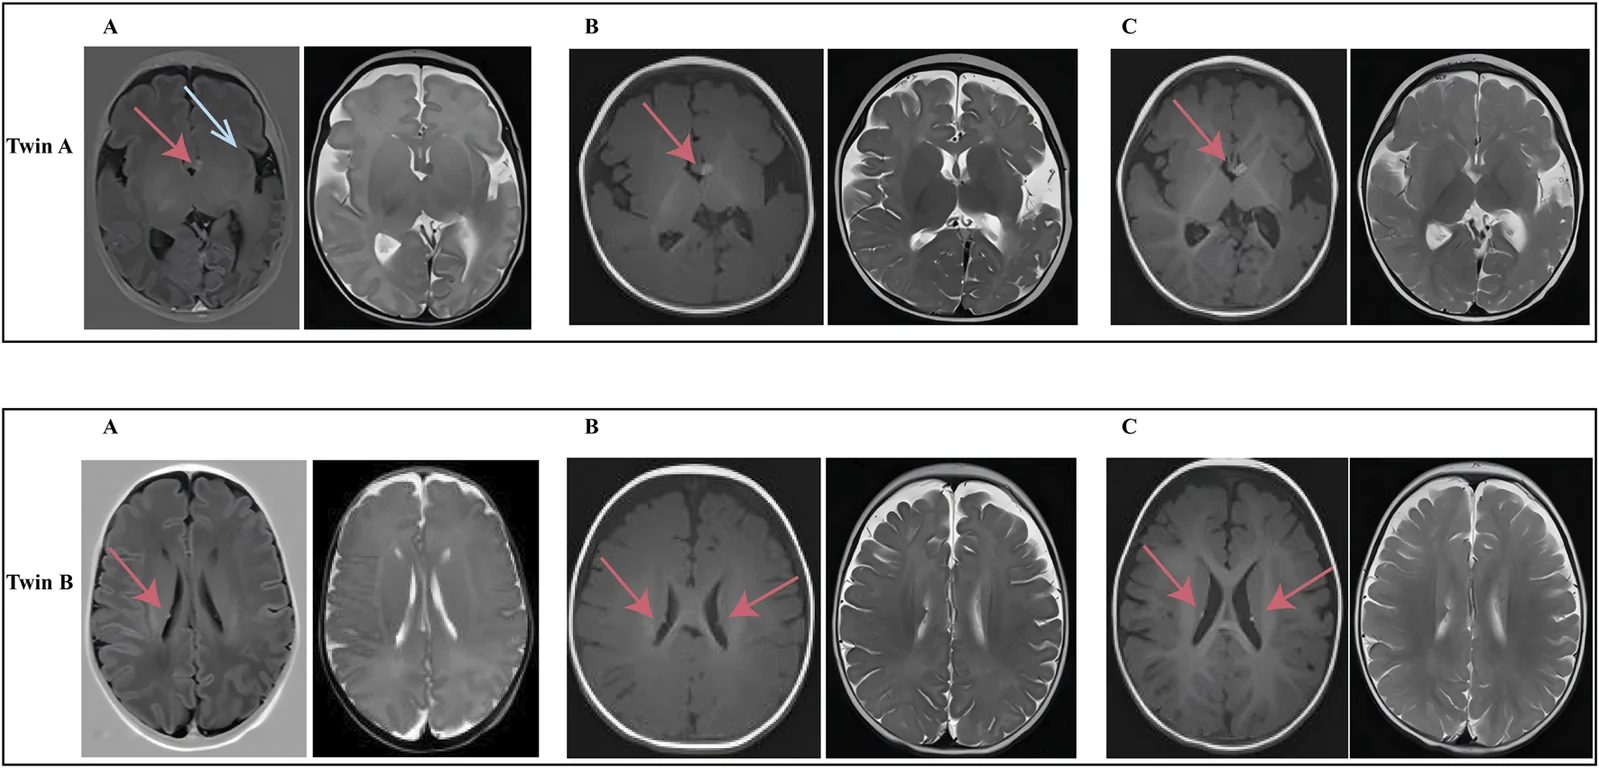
Serial brain MRI findings in a preterm twin with co-occurring SMA and TSC. (A) Baseline MRI at 6 weeks of age; (B) Follow-up MRI at 6.5 months of age; (C) Follow-up MRI at 12 months of age. For each patient and time point, the left and right images show T1-weighted and T2-weighted sequences, respectively. In Twin A, the blue arrow indicates a stable polymicrogyria-like cortical malformation, whereas the red arrows indicate left subependymal nodules (SENs), which increased slightly in number and size at 6.5 months and remained visible at 12 months. In Twin B, red arrows indicate a right SEN at baseline and a newly identified left SEN at 6.5 months; bilateral SENs remained stable at 12 months.
